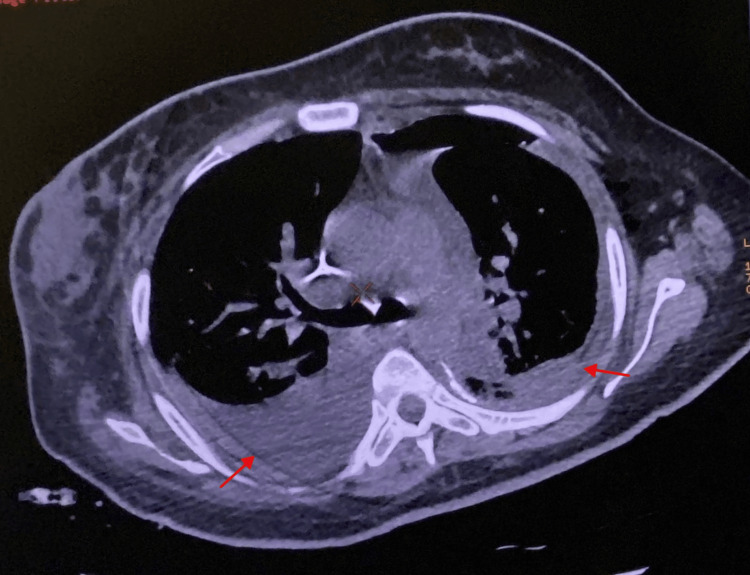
CT scan showing bilateral pleural effusions.
